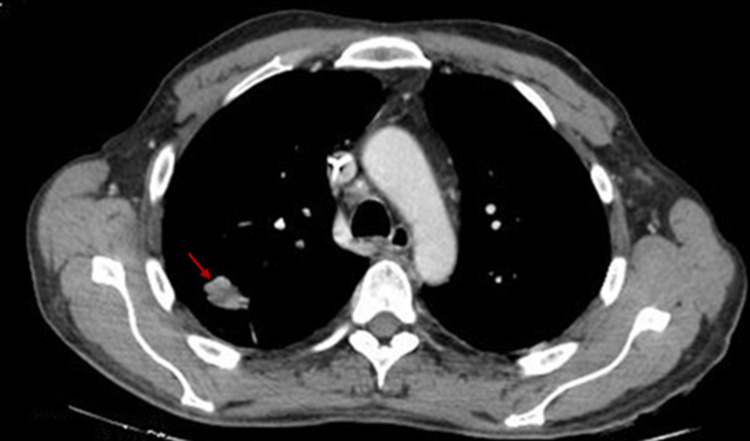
Axial contrast-enhanced chest CT scan. Axial CT image of the thorax showing a right upper lobe mass with soft tissue and intermediate density, located in the posterior segment. The lesion has irregular and microlobulated contours and measures approximately 22 × 13 mm.
